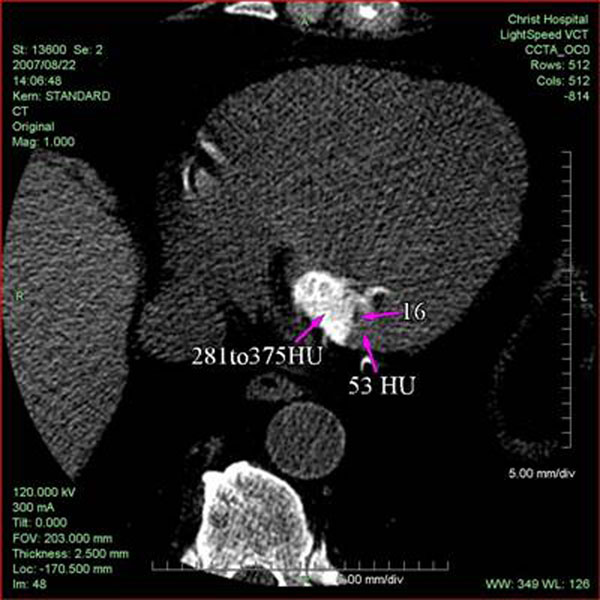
High resolution thin section CT axial view
